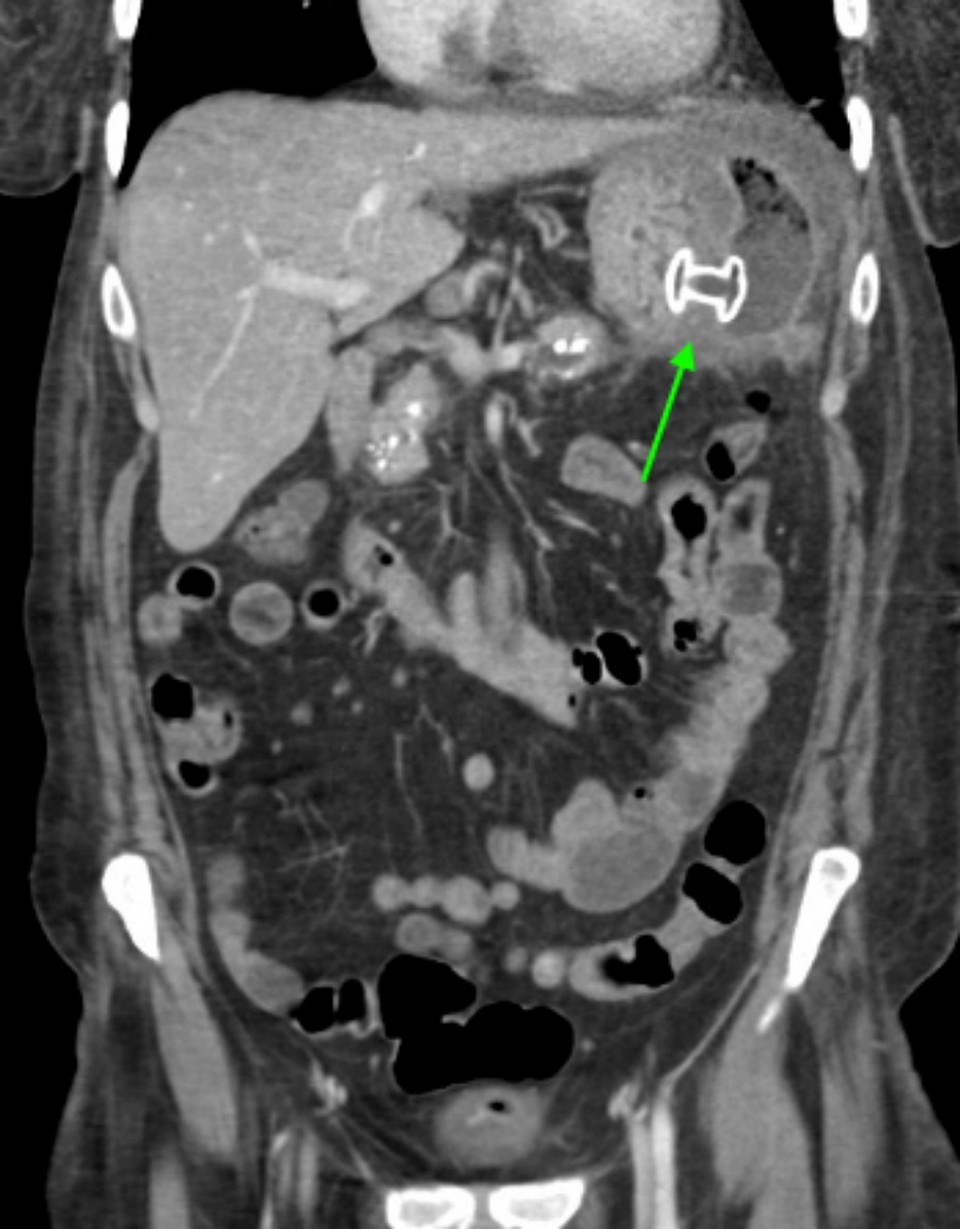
Coronal view CT of the abdomen and pelvis with intravenous contrast post cystogastrostomy stent (arrow) placement demonstrating decompression of the giant pseudocyst. Residual gas and fluid are present in the collection. CT - computed tomography
